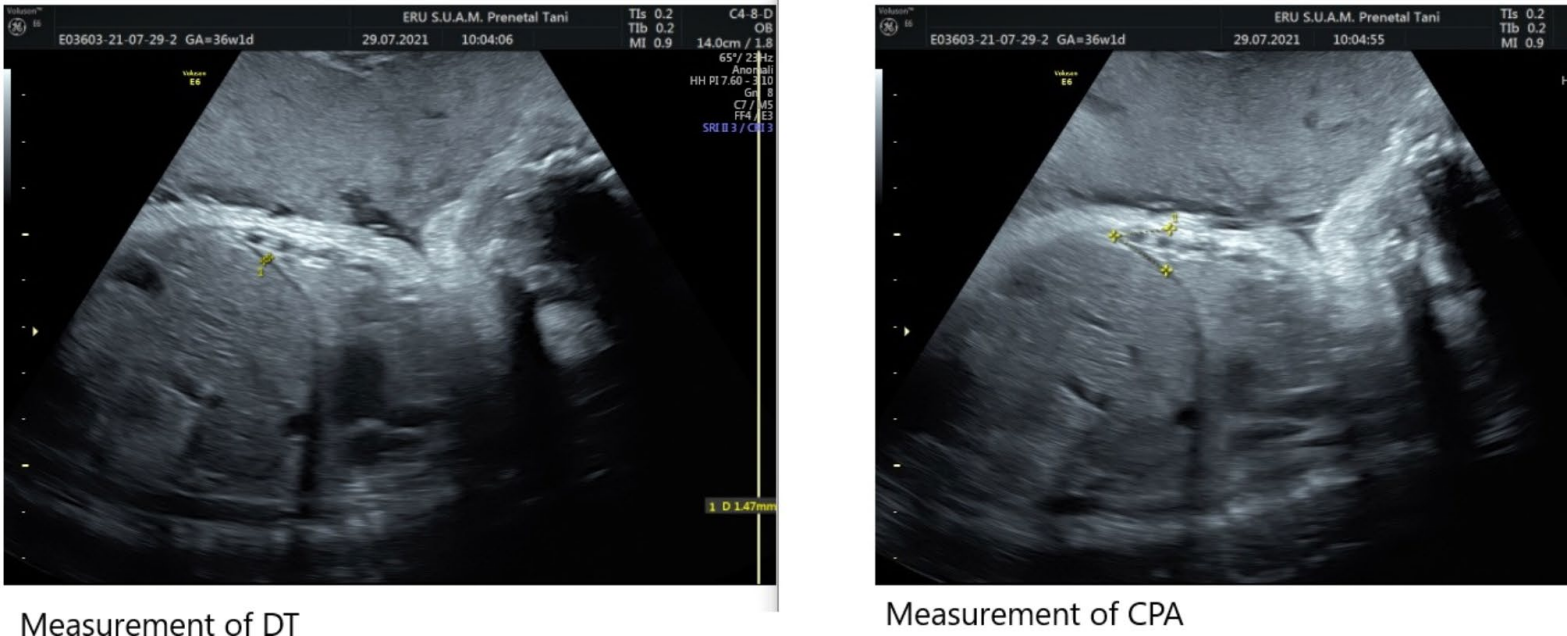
Ultrasonographic measurement of diaphragm thickness and costophrenic angle
